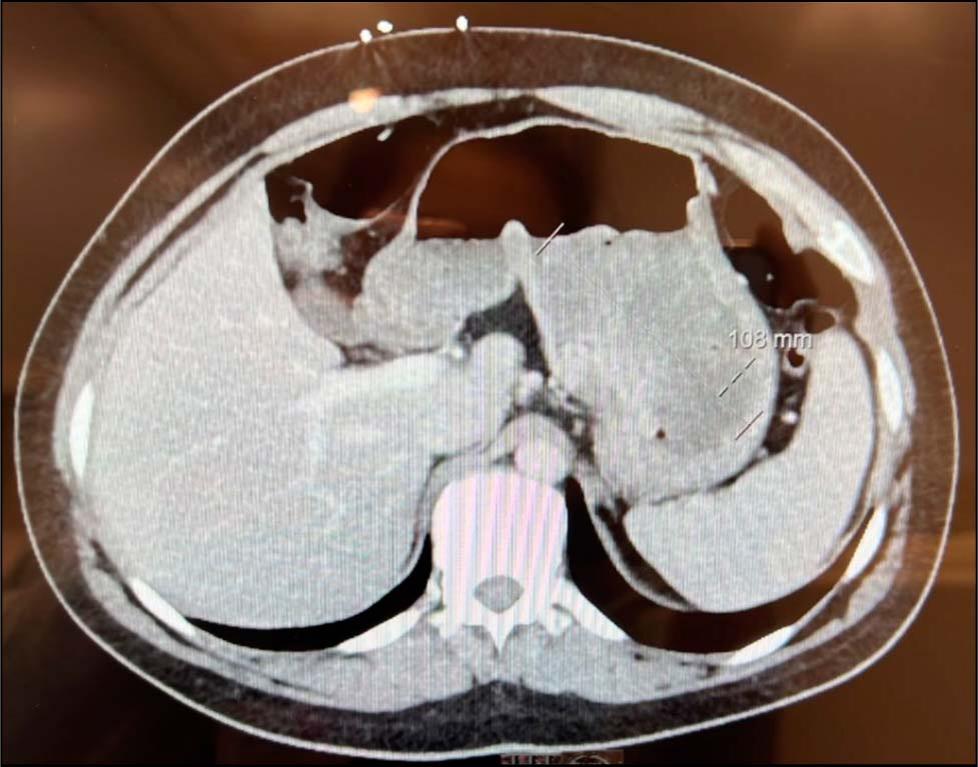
Computed tomography scan of the abdomen with contrast reveals a polypoid mass along the gastroesophageal junction, moderate diffuse gastric wall thickening of the fundus, and proximal body of the stomach.
